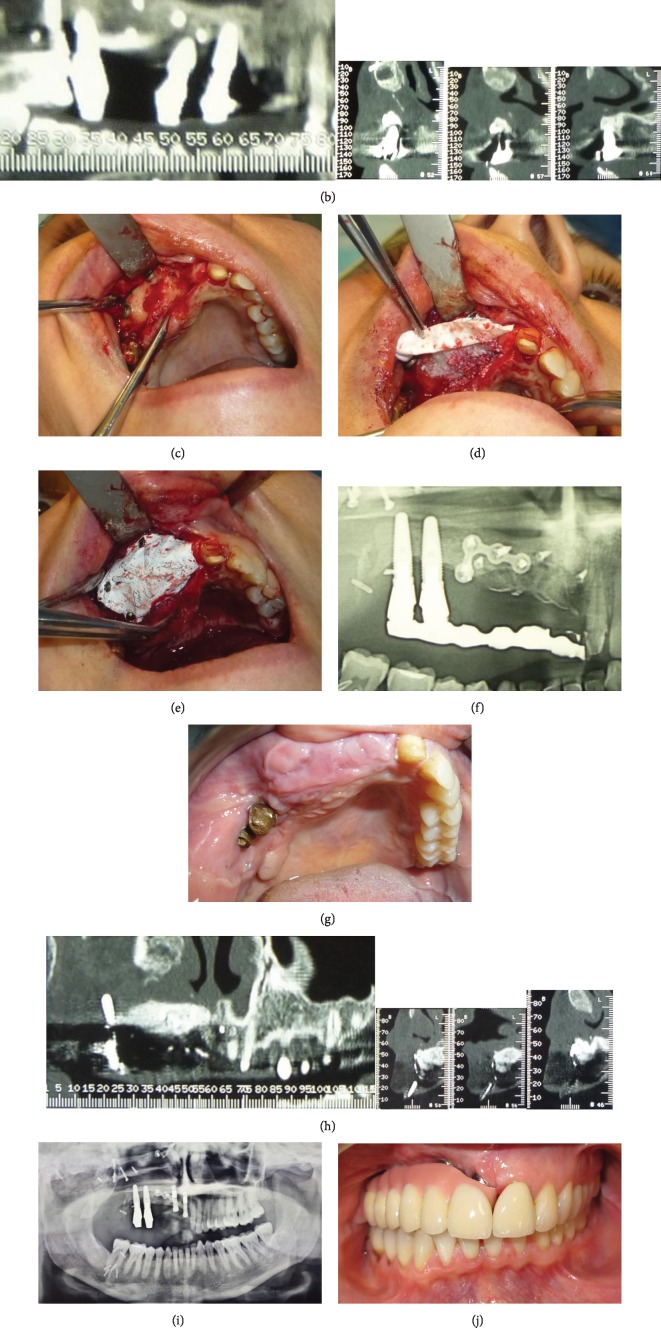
(a) Clinical image shows peri-implantitis, hypertrophy, and severe gingival inflammation. (b) Computerized tomography scan. Preoperative view showing insufficient bone in the apicocoronal and buccolingual dimension to place implants according to the prosthetic needs. (c) Clinical image showing bone defect. (d) Clinical image prior to fixing the membrane on the palatal side showing the graft that completely covers the defect. (e) Clinical image with the membrane completely in place. (f) Panoramic radiograph post-GBR. (g) Clinical image shows tissue healing at three months. (h) Computerized tomography scan. Postoperative view showing robust bone regeneration in the area corresponding to the radiopaque marks on the surgical stent. (i) Panoramic radiograph postimplant surgery. (j) Clinical view of the implant-supported fixed prosthesis. Frontal view. (k) Clinical view of the implant-supported fixed prosthesis. Maxillary occlusal view.
